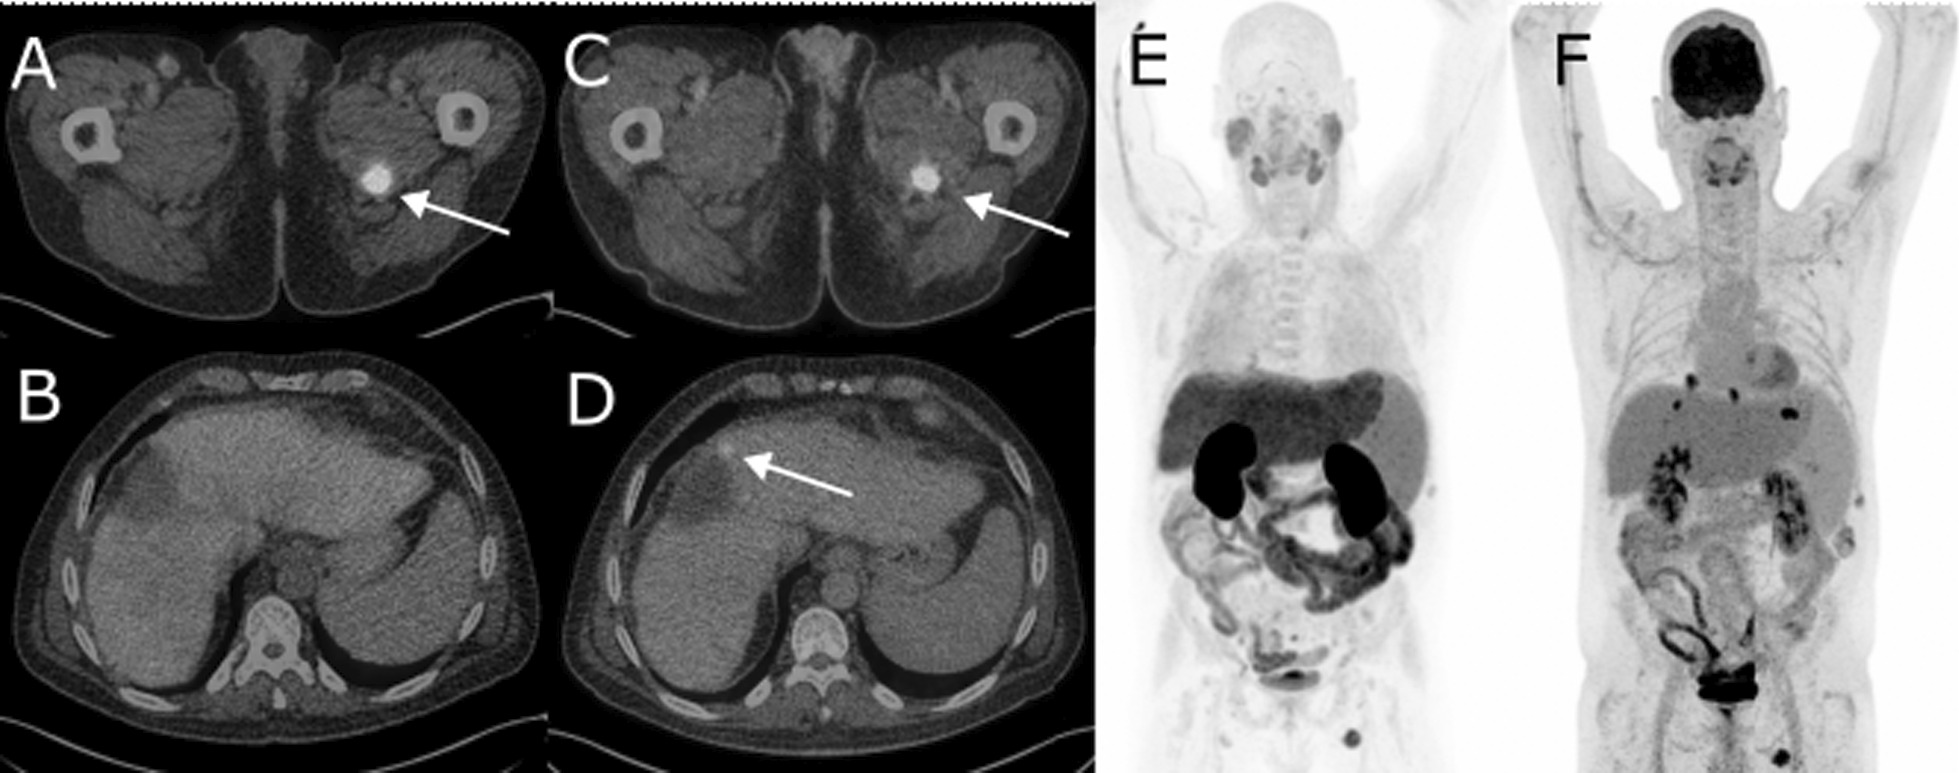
PET/CT imaging of a 49-year-old male patient (#1) known with hemochromatosis-related cirrhosis and multifocal HCC. Underwent MWA of solitary HCC node (32 mm) in segment VIII to ensure eligibility for orthotopic liver transplantation (OLT). Post-therapy MRI follow-up at three months displayed further progression of several intrahepatic lesions and potential tumour thrombus in v. porta, which prompted dual-tracer diagnostic with [18F]FDG and [11C]CH PET/CT. Both tracers found a metastasis in the m. adductor longus muscle (A for [11C]CH, and C for [18F]FDG, arrow), while an ablation site recurrence was only found by [18F]FDG (D) and not by [11C]CH (B). In addition, MIP images show several mediastinal lymph nodes only found by [18F]FDG (F), whereas [11C]CH (E) has physiological uptake in the same region. Due to lymphatic and extrahepatic disease patient received palliative chemotherapy
